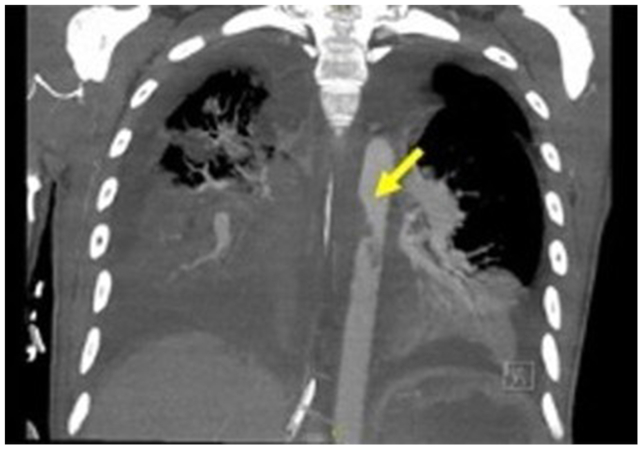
Initial computed tomography scan with aortic irregularity.
